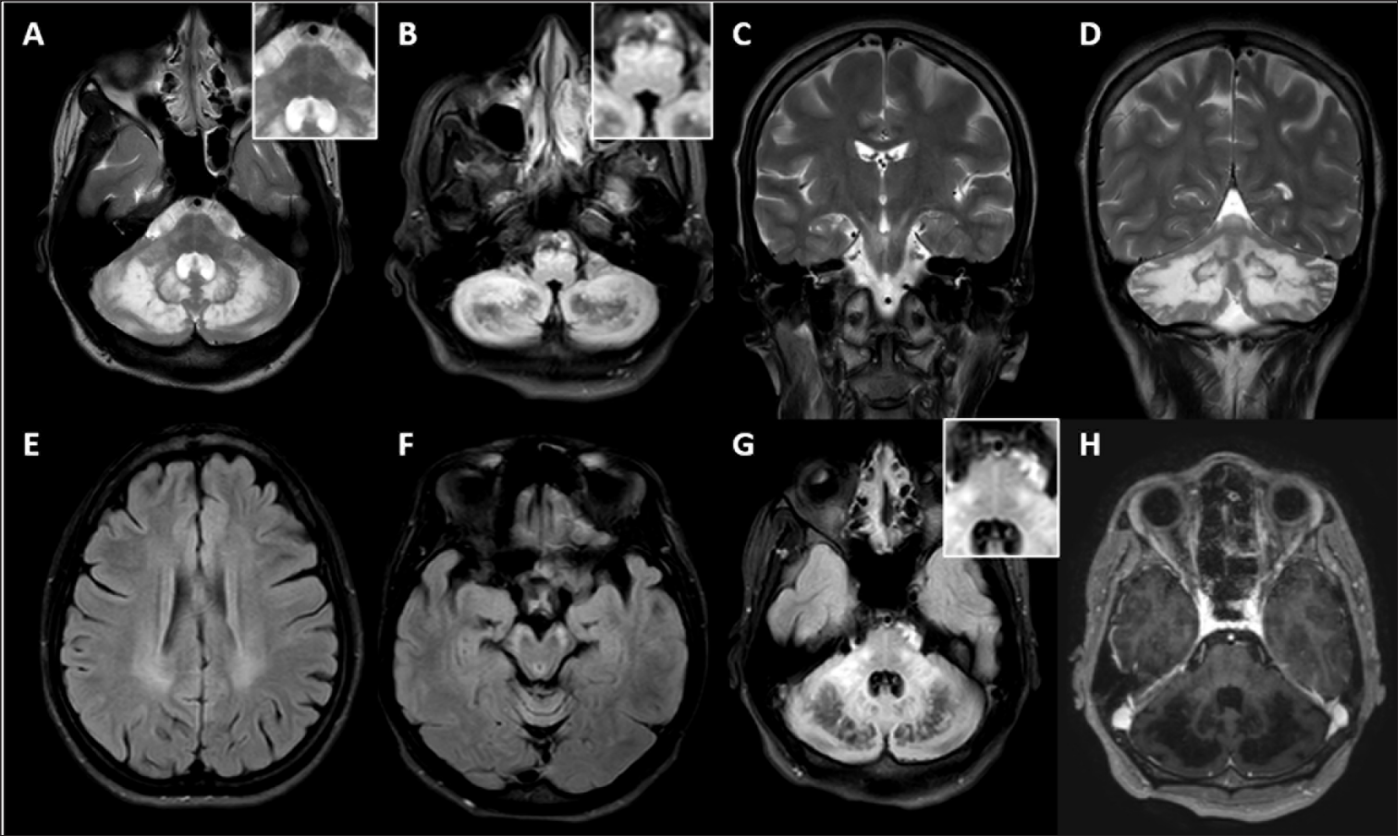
MRI of Patient-1. T2 (A, C, D), FLAIR (B, E-G) and post contrast T1 (H) sequences of MRI brain of Patient-1 showing symmetric T2 hyperintense and FLAIR hypointense lesions along bilateral dentate and cerebellar white matter (A, B, D, G), T2 and FLAIR hyperintensity of corticospinal tract along periventricular white matter (E), posterior limb of internal capsule (C), cerebral peduncles (F), pons (A and H), inferior olives (B), middle cerebellar peduncles (A and G) and rarefaction along cerebellar white matter (A and G). T2 hypointense rim noted around dentate nuclei (D). Note the “Hot cross bun” appearance along the pons (A and G, inset) and olivary hyperintensity (B, inset). T1 post contrast image showed no enhancement (H).
